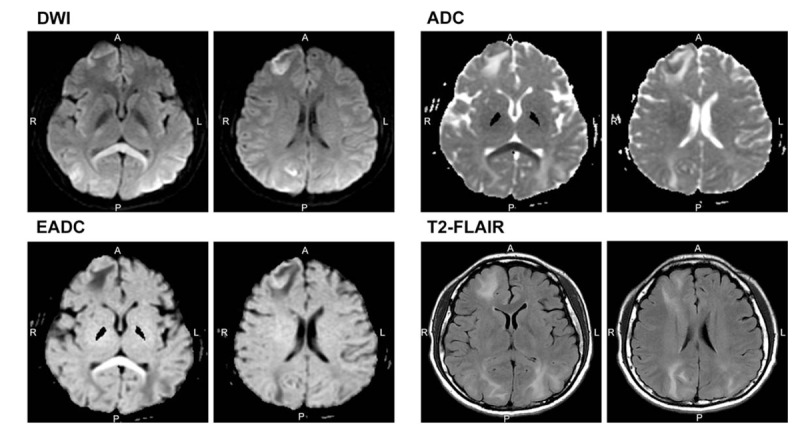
Brain magnetic resonance imaging (MRI) shows high-signal intensity lesions on diffusion-weighted imaging (DWI), exponential apparent diffusion coefficient, and T2 fluid-attenuated inversion recovery (T2-FLAIR) images in the right frontoparietal, temporal, occipital lobes and left temporal, parietal, and occipital lobes, splenium of the corpus callosum with gyral enhancement. Apparent diffusion coefficient (ADC) series also show high-signal intensity in the aforementioned cortex area. However, low-signal intensity is observed in the right frontal area and the splenium of the corpus callosum, indicating cytotoxic edema at these 2 areas.
